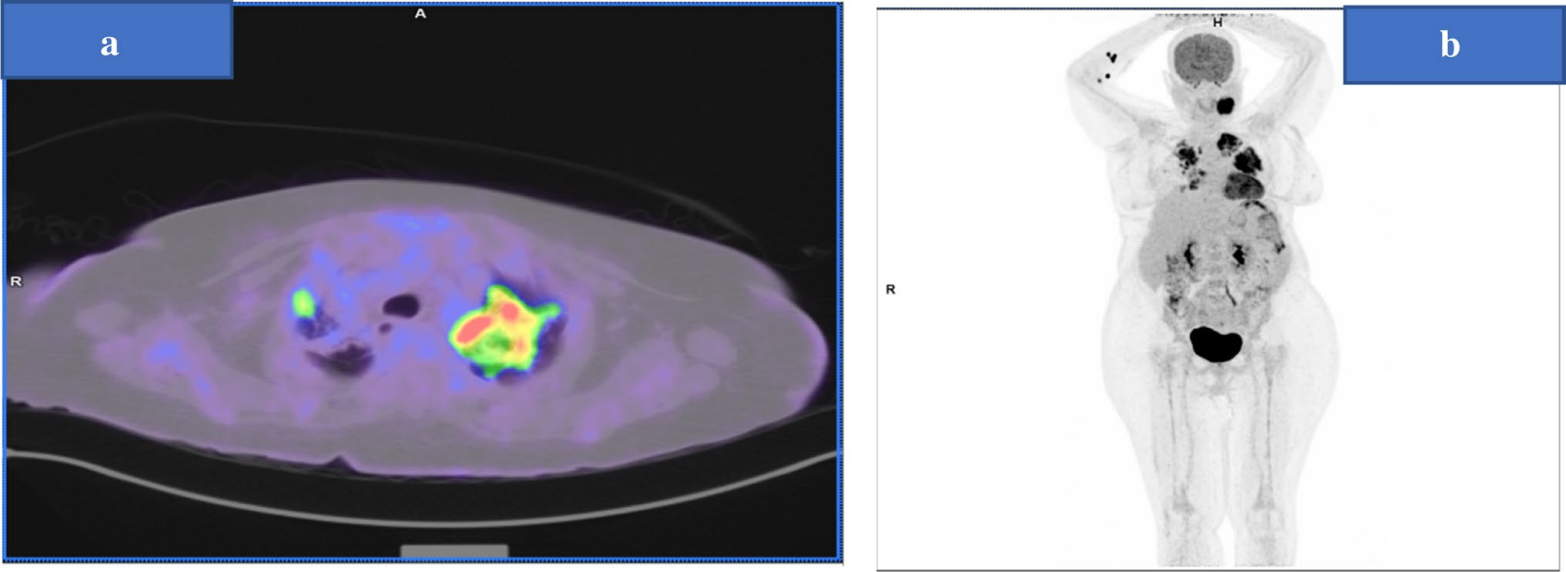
a Fused FDG PET-CT image with lung window showing intense uptake bilaterally more prominent in the left apical mass. b maximum intensity projection representation of whole body FDG distribution showing metastatic pulmonary adenocarcinoma (stage IV)—note FDG avidity is more on left side
